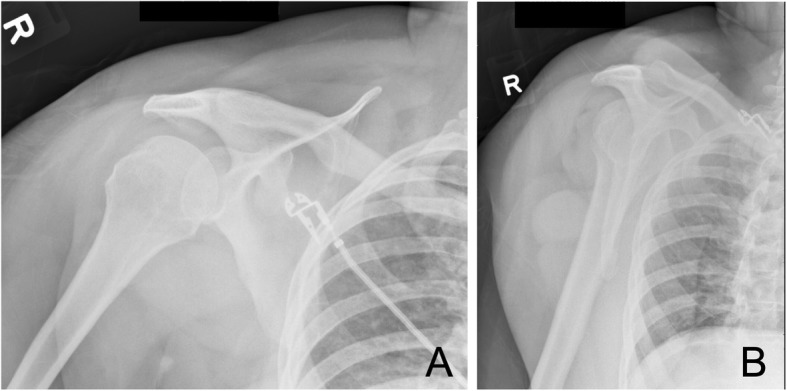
Post-reduction plain radiographs. a AP view of the right shoulder following reduction. b Scapular Y view of the right shoulder following reduction
